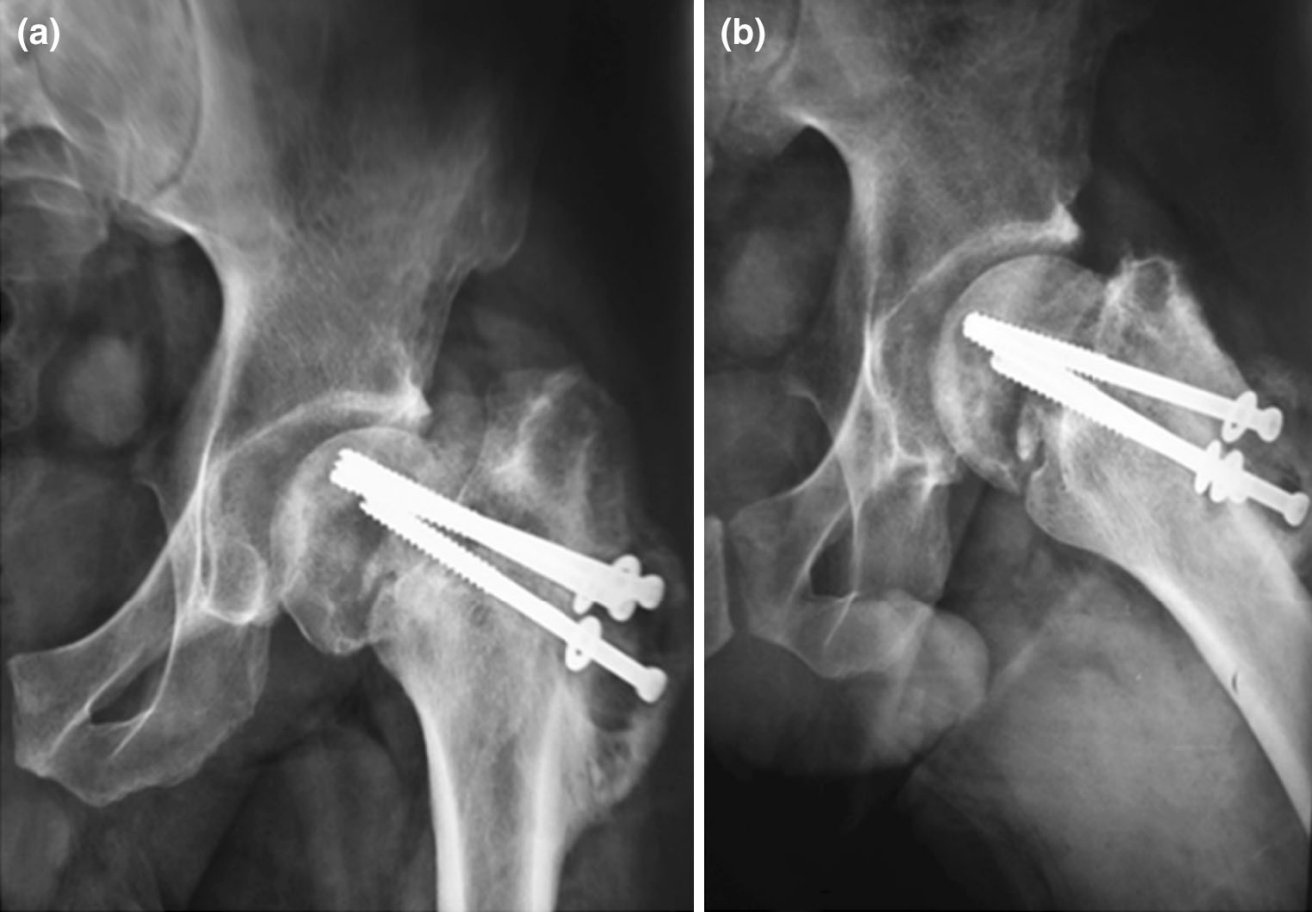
Anteroposterior (a) and lateral (b) X-rays of the hip in a case of infected non-union of intracapsular fracture showing collapse of the femoral neck in addition to radiological manifestations of infection (osteolysis around the screws and periosteal reaction)
